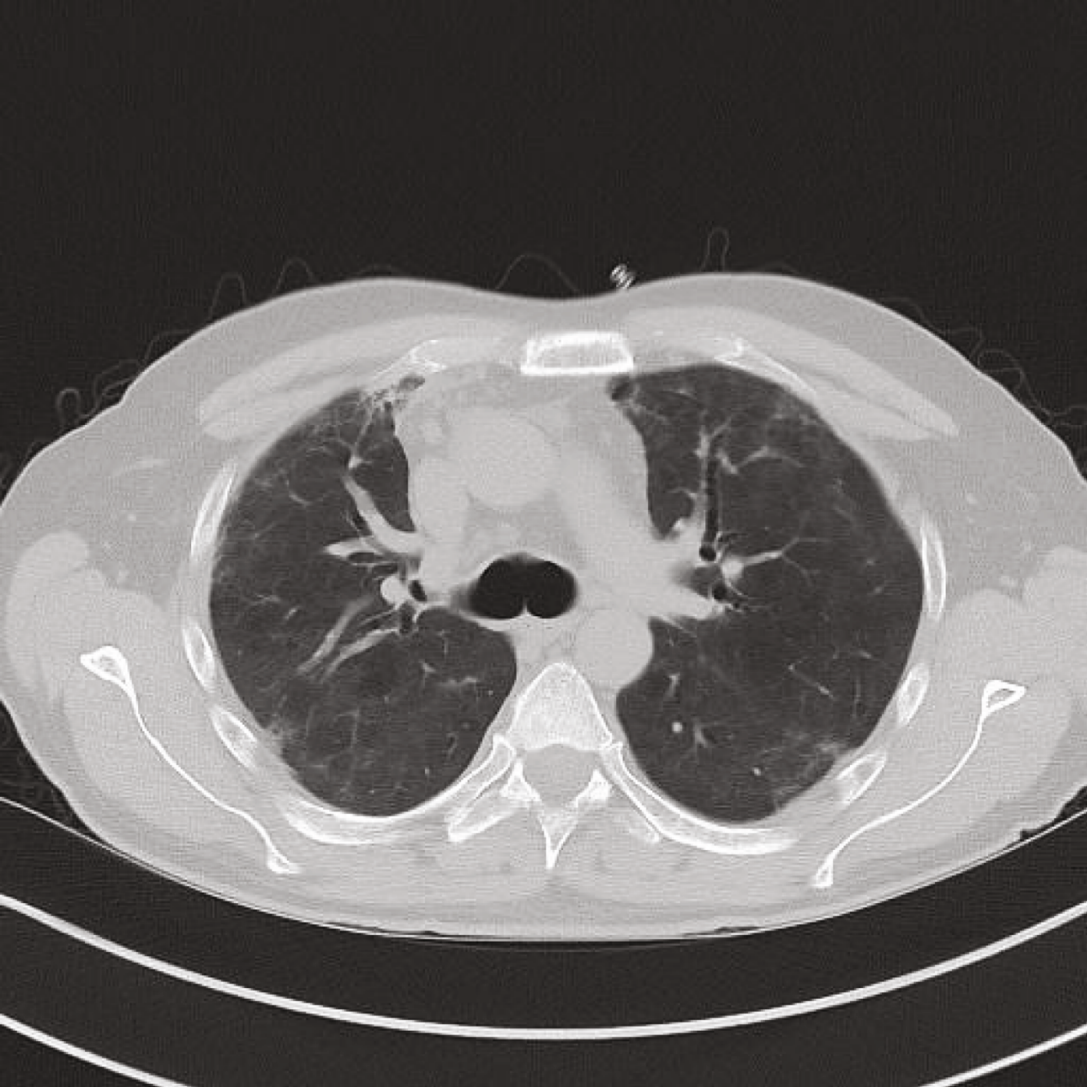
Chest CT-scan 31 days after ECMO implantation, the same level as Figure 1. The pneumothorax and pneumomediastinum completely abated, and the ground glass opacities profoundly receded.
